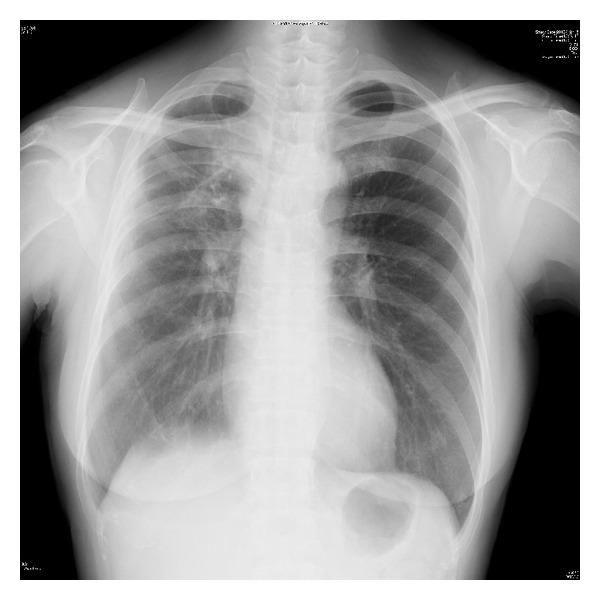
Chest X-ray (PO 4 months). A chest X-ray reveals an improved clear field in the right lung.
